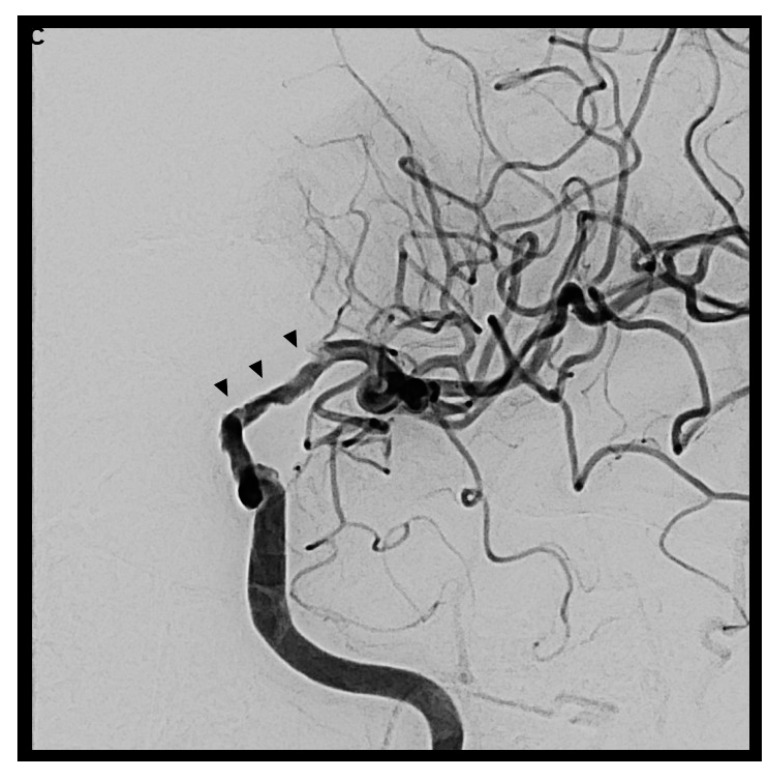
Diffusion-weighted imaging in cerebral MRI showed now new areas of ischemia within the middle cerebral artery territory (A). Further evaluation with cerebral MR Angiography (B) revealed new, compared to earlier imaging a few days before, irregularities in the vessel walls of the left intracranial internal carotid artery, with moderate-to-severe luminal narrowing. To clarify these findings, Digital Subtraction Angiography (DSA) was performed (C). The DSA indicated that while the extracranial segment of the left internal carotid artery appeared normal, significant irregularities were present beginning at the petrous segment. These irregularities (black arrows) caused moderate luminal narrowing with partial filling of the middle cerebral artery from crossflow via the A1 segment. During the venous phase, adequate contrast filling of the cavernous sinus was observed, effectively ruling out a cavernous sinus thrombosis. Despite local administration of 3 mg Nimodipine over 30 min, there was no alteration in the observed vascular irregularities, supporting the conclusion that these changes were inflammatory rather than spasmodic.
